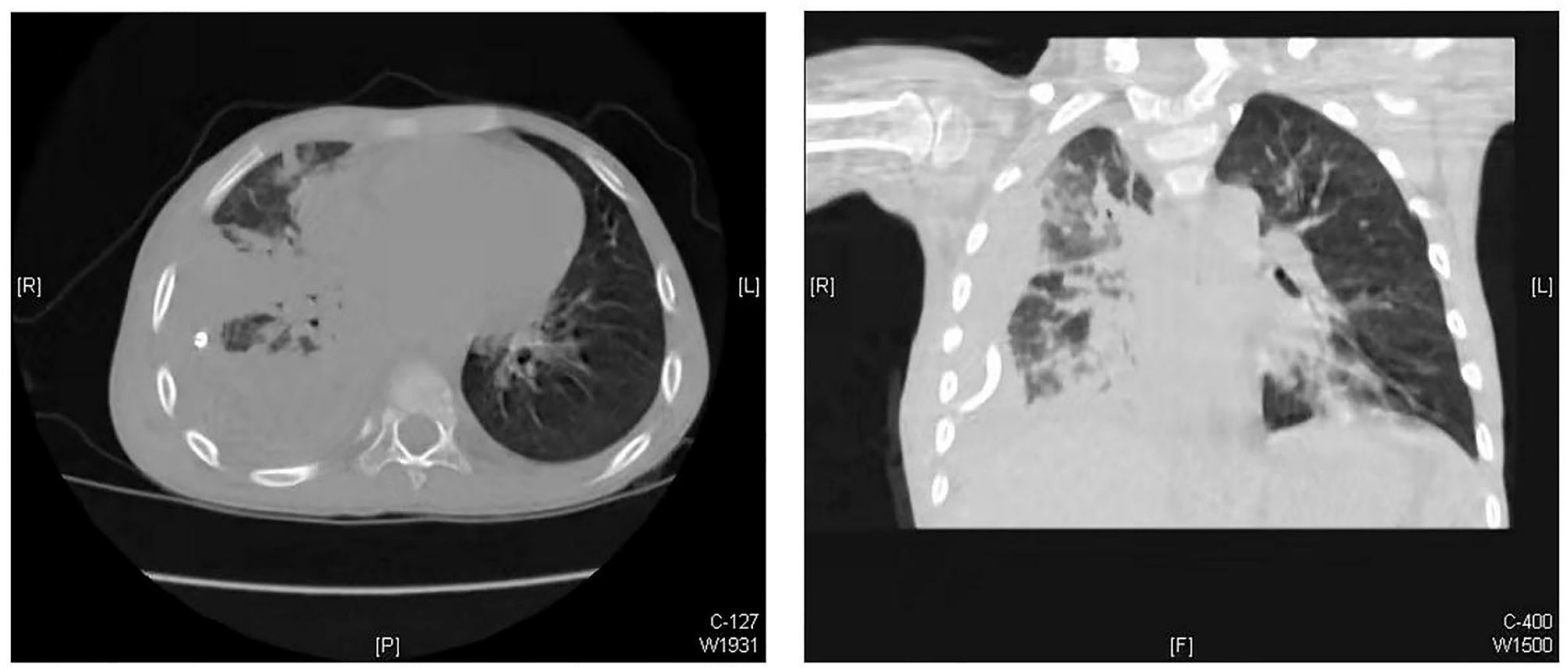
CT image of pleural empyema.
